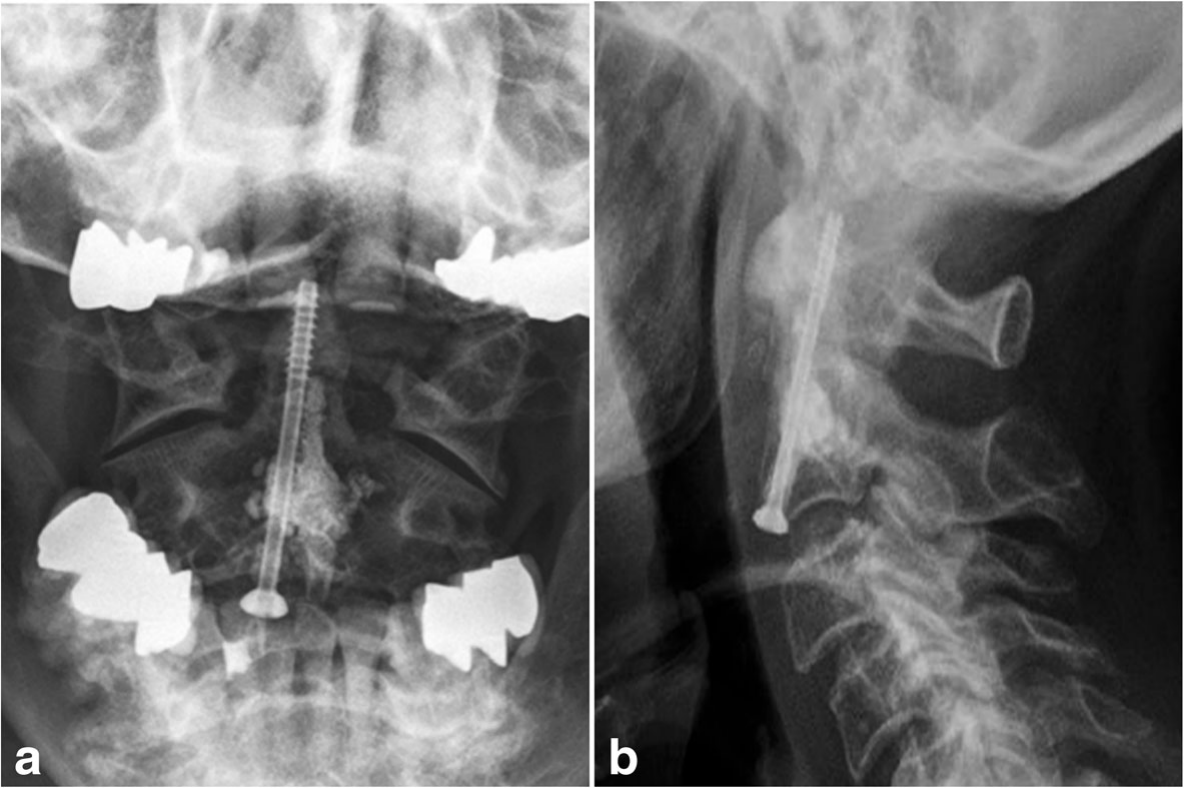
X-ray of the dens (a) and the lateral (b) cervical spine of the first patient shows favorable cement filling of the osteolytic lesion, in the base of the dens and the additional anterior odontoid screw (Case 2)
